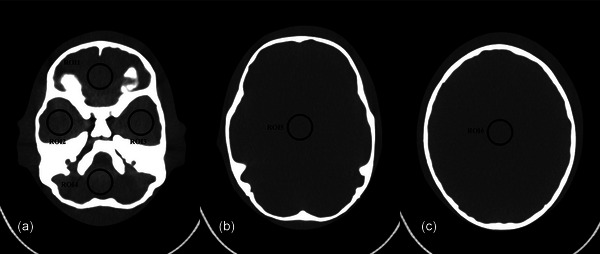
ROIs for quantitative analysis of image quality. ROI 1: Anterior cranial fossa; ROI 2: Right middle cranial fossa; ROI 3: Left middle cranial fossa; ROI 4: Posterior cranial fossa; ROI 5: Central basal ganglia region; ROI 6: Central corpus callosum trunk. CT, computed tomography; ROI, region of interest.
